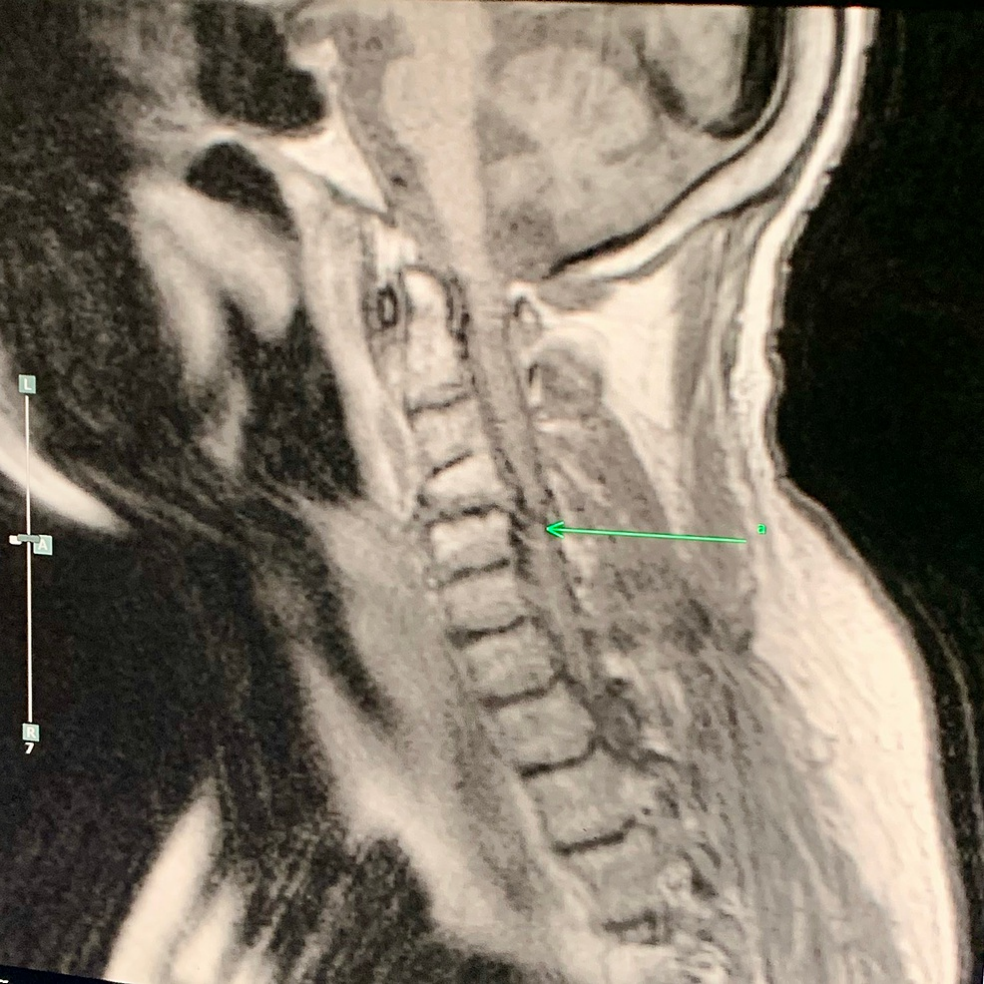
MRI of the cervical spine showed large disc herniation with congenital cervical canal stenosis
